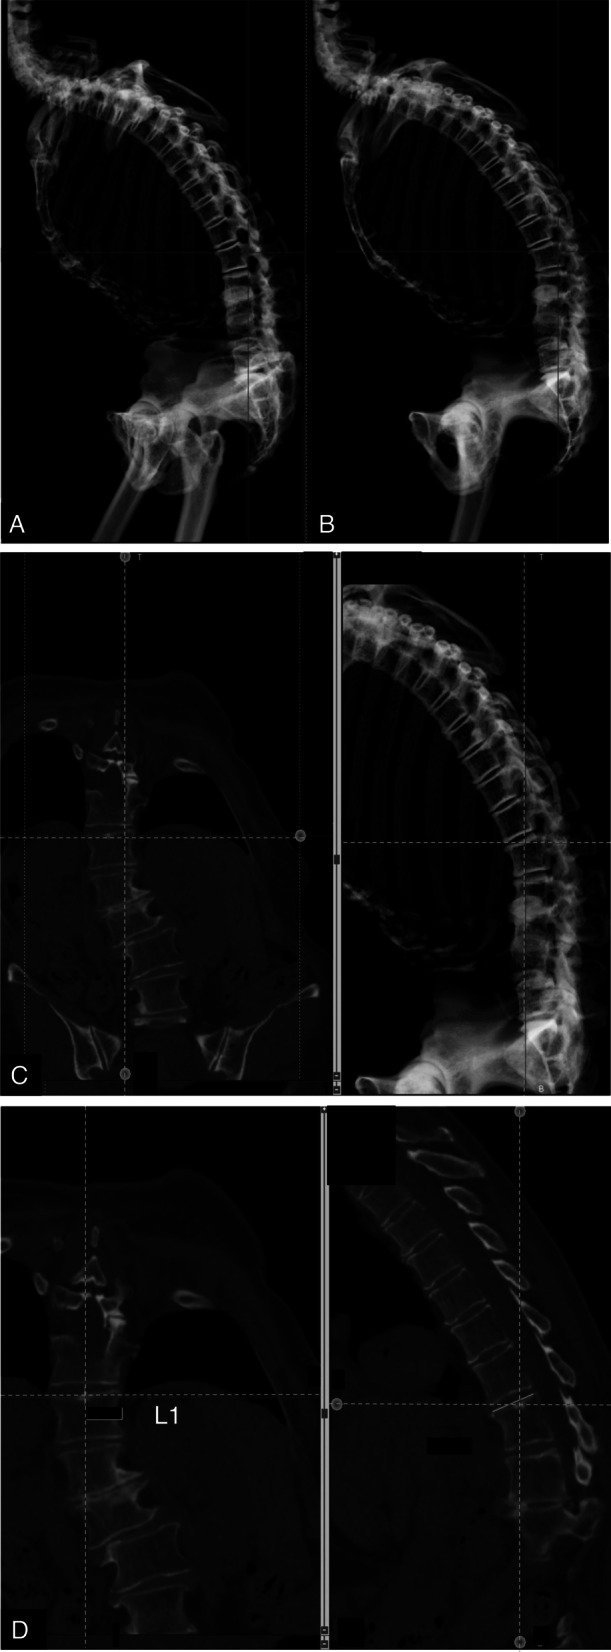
Example of measuring the tilt of sagittal plane of L1 upper endplate. A,B Rotate axially in DRR image from A to B, and overlay bilateral femoral head. C Left: CT image. Right: DRR image. Select the center of the target endplate in the DRR image and obtain the coronal image on that line. (Reference lines are linked between DRR image and CT image.). D left: Select the center of the left and right vertebral bodies with the coronal image and configure the sagittal plane. Right: Sagittal CT image use for measurement and L1 upper endplate tilt. (Reference lines are linked each CT images)
